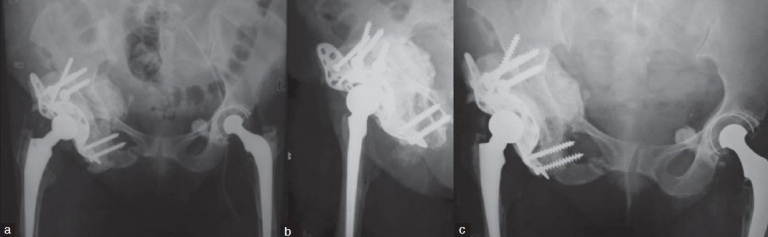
(a) Early postoperative X-rays of the pelvis including both hip and thighs (anteroposterior) and (b) lateral view of the right hip with thigh showing a well reconstructed acetabular defect using total acetabular allograft, morselized bone graft and Burch-Schneider reconstruction cage. (c) One-year follow-up X-rays of pelvis including both hips (anteroposterior view) showing graft union and incorporation
